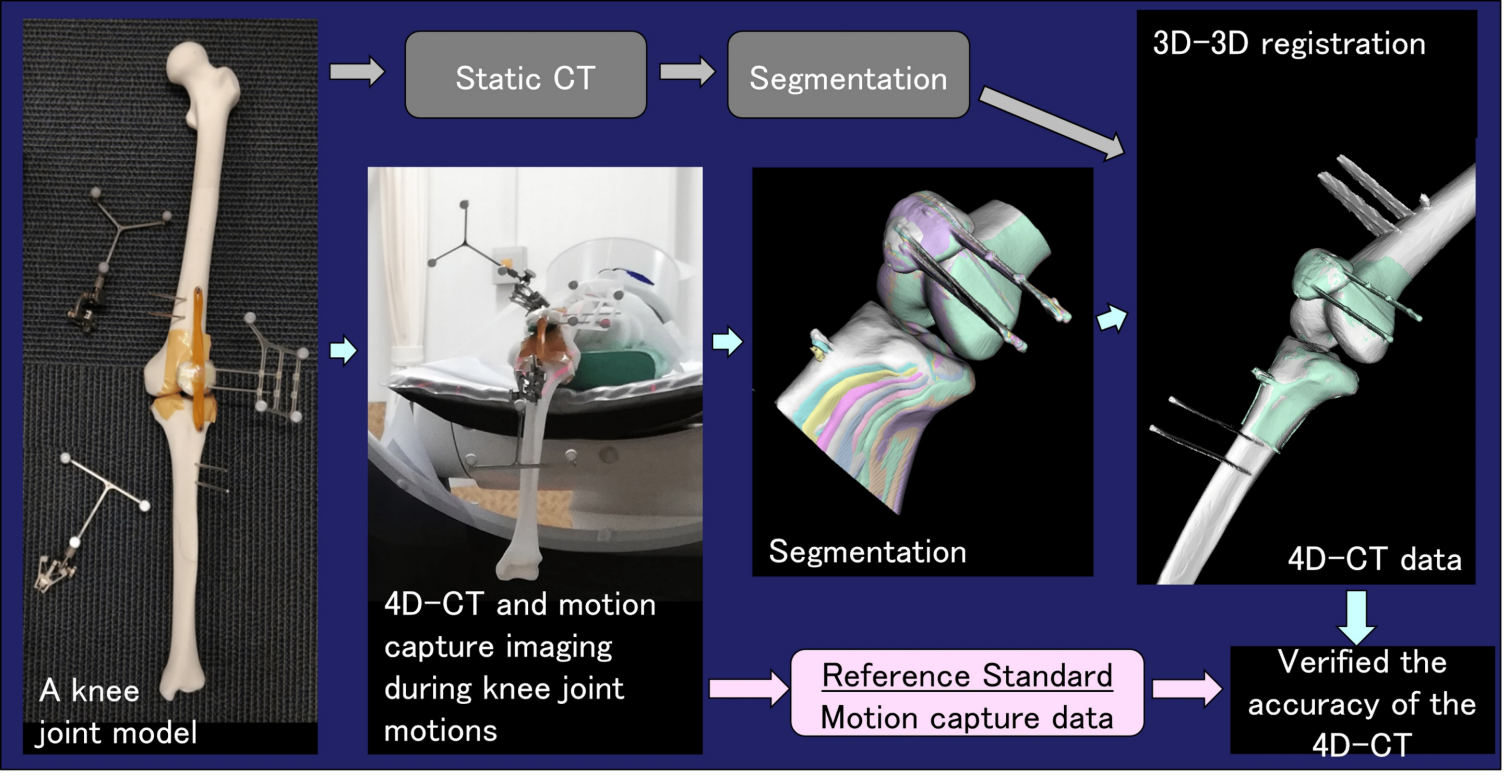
Process flow from CT acquisition to dynamic analysis Static of the entire knee joint model and 4D-CT of the knee joint motion were acquired, and 3D-3D registrations were performed between them to measure the position and posture of the knee joint motion from the 4D-CT. The position and posture of the knee joint motion were measured simultaneously using the optical-motion capture system during the 4D-CT scans were performed, and the results were used as a reference standard. The accuracy of the 4D-CT was verified by comparing the positional orientation measured by the 4D-CT with the reference standard.
